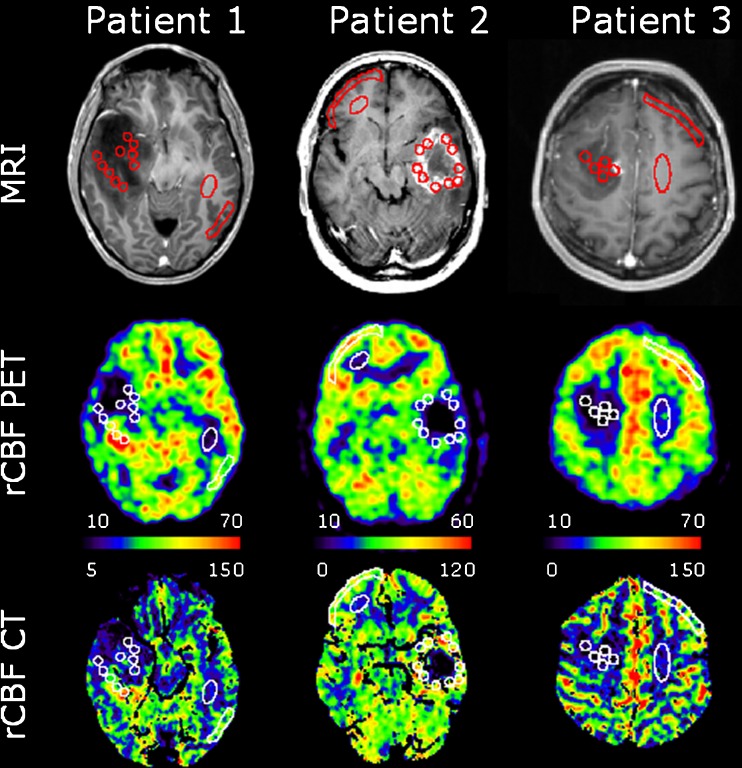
Patients with coregistered transaxial slices of post-contrast preoperative T1-weighted MRI, rCBF PET and PCT scans (ml min^−1^ 100 ml^−1^) documenting the locations of ROIs. Highly vascular areas are eliminated in PCT. Patient 1 suffered from a heterogeneous, non-enhancing astrocytoma (grade II) with central hypoperfused and posterior hyperperfused areas. Patient 2 had a cystic ring-enhancing moderately perfused glioblastoma multiforme (grade IV). Patient 3 had an enhancing uniformly hypoperfused astrocytoma (grade II). Note the different scaling of rCBF PET and rCBF CT images. The *top row* shows T1-weighted MR images with gadolinium contrast
